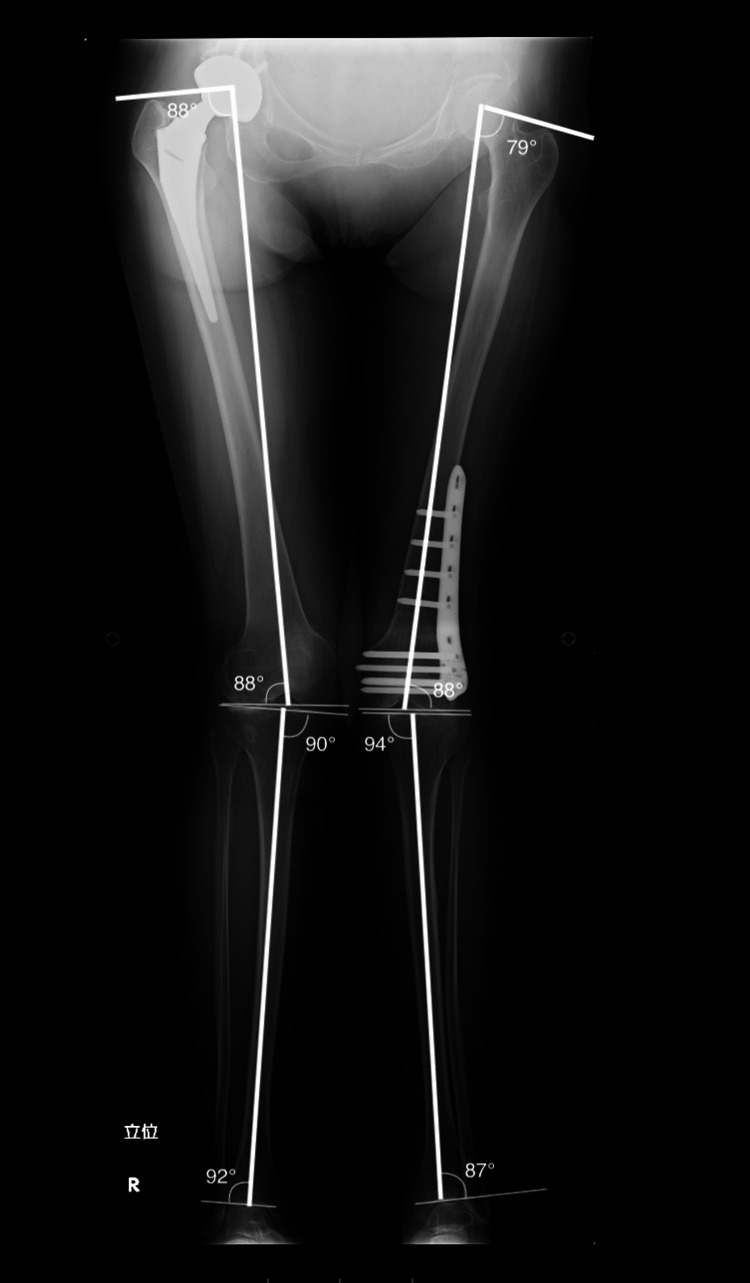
Postoperative Measurement of Alignment Parameters Postoperative Radiograph of the entire lower limb with Coronal alignment parameters.
